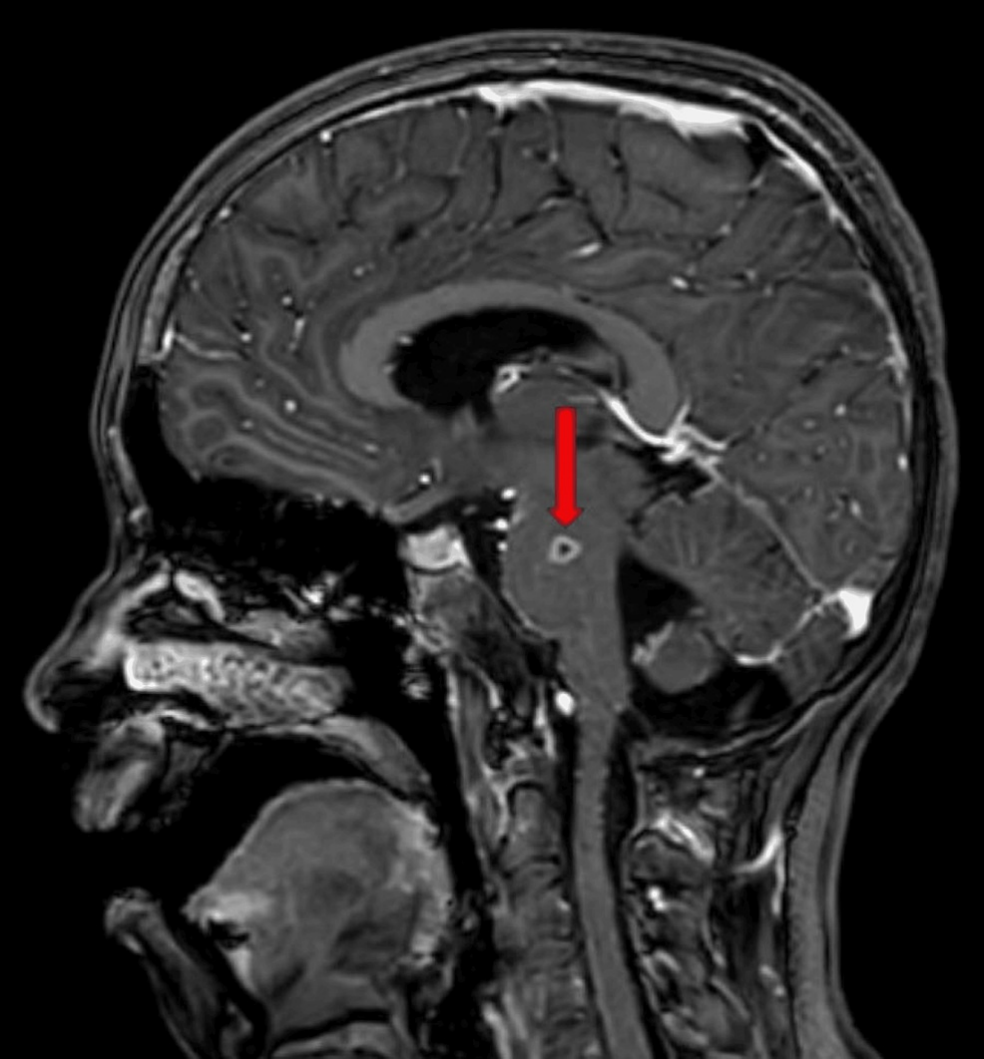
A sagittal section of magnetic resonance imaging showing a peripherally enhancing altered signal intensity lesion in the pons (red arrow).
